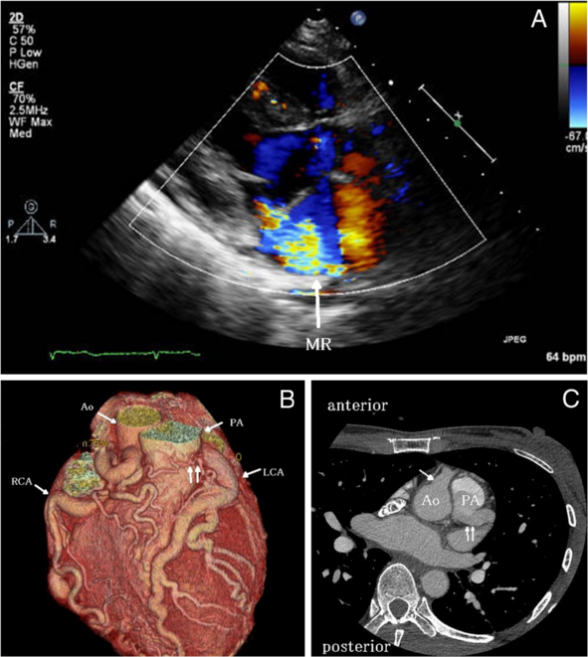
Results of diagnostic examination. (A) Long-axis view on preoperative transthoracic echocardiography showing moderate or severe mitral regurgitation. (B) Preoperative multidetector-row computed tomography. The RCA and the LCA were dilated, and collaterals between the two coronary arteries were observed. (C) Preoperative axial image obtained via enhanced computed tomography. The arrow indicates the RCA orifice, and the double arrows indicate the LCA orifice. The LCA originated from the PA. The orifice of the LCA was located 1.8 cm from the Ao. Ao, aorta; PA, pulmonary artery; RCA, right coronary artery; LCA, left coronary artery.
